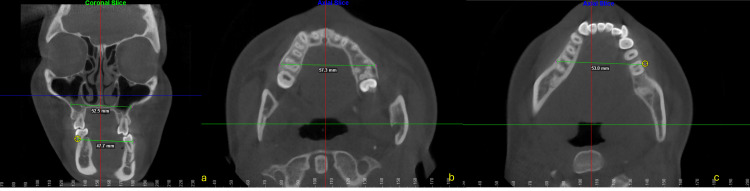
Tomographic slices in different planes obtained using cone-beam computed tomography (CBCT). a. Coronal slice: The relationship between the upper dental roots and adjacent anatomical structures is shown. Transverse measurements of the maxilla at the level of the first molars are highlighted, with distances. b. Axial slice (maxilla): The upper arch is visualized in the axial plane, showing an interpremolar/intermolar transverse distance. c. Axial slice (mandible): The lower arch is observed in the axial plane as well, with a transverse measurement of 53.0 mm between the lower molars.
